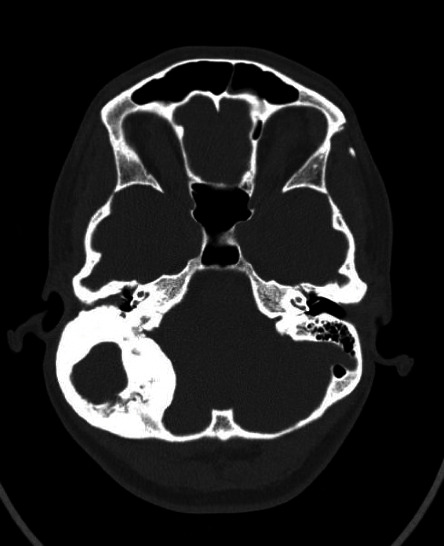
Initial computed tomography scan showing right bony thickening, sclerosis, and expansion centered around right posterior petrous temporal bone with extension into the posterior external auditory canal with central lucent structure. There is compression of the right posterior fossa.
